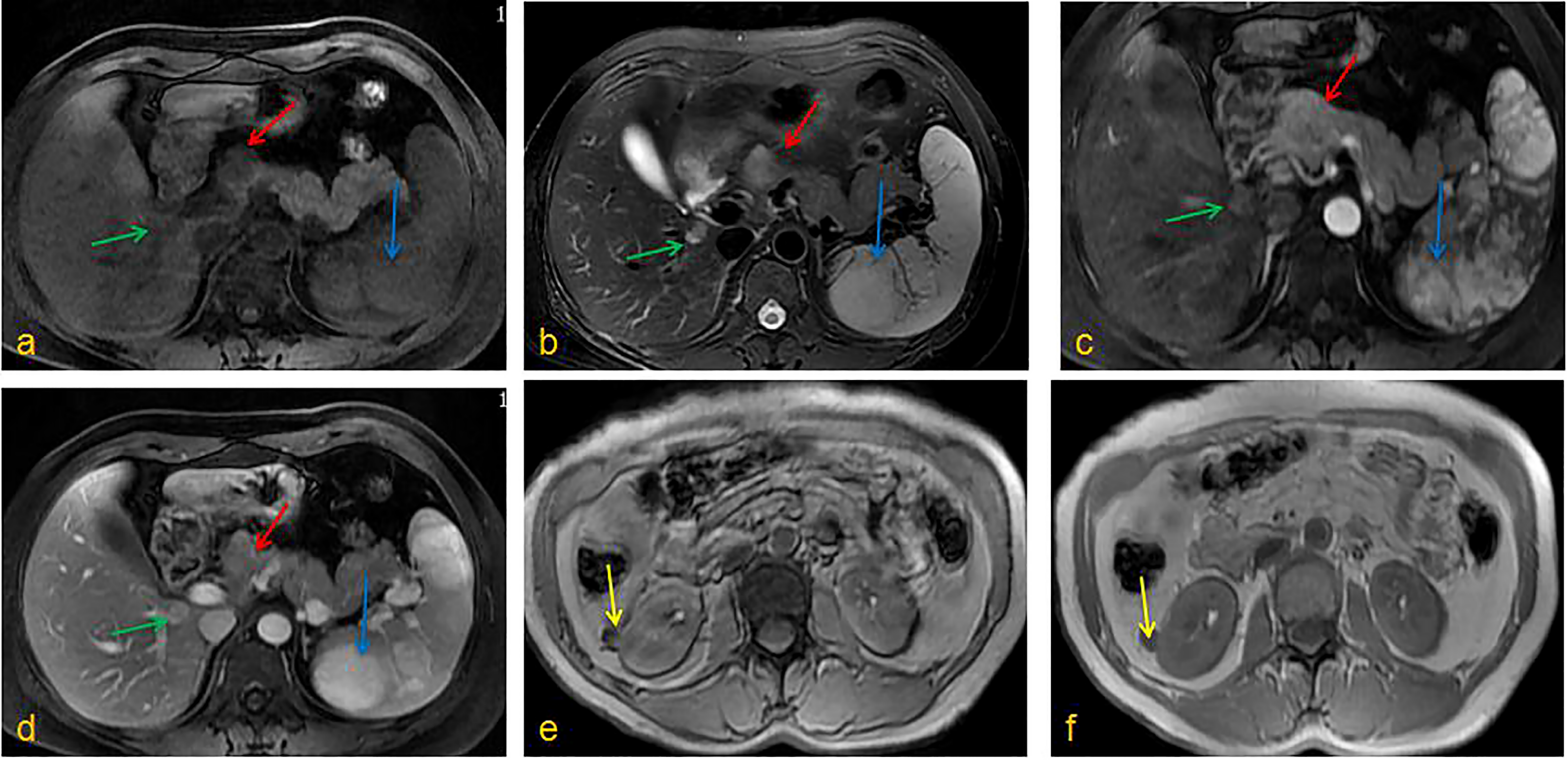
Enhanced MRI of the abdomen. (A) T1WI, (B) T2WI, (C) Enhancement phase, (D) Delay phase: the pancreatic head could be seen a clear outline of about 2.5cm in diameter with long T1T2 signal, the lower segment of the right liver had oval shadow of about 1.4cm in diameter with long T1T2 signal, the spleen showed multiple irregular T1 signals and long T2 signals; (E) T1WI, (F) T2WI, kidneys could be seen round T1 signal and low T2 signal foci, and the enhanced arterial phase showed obvious enhancement. (red arrow: pancreatic tumor; blue arrow: splenic tumor; green arrow: liver tumor; yellow arrow: kidney tumor).
